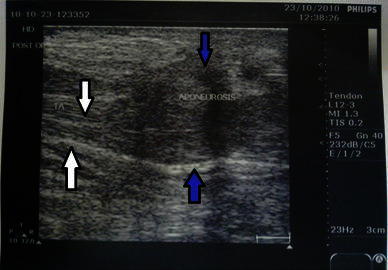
Ultrasound scan after 6 months showing continuation of fibers at the repaired site. The *blue arrows* show the repaired apenurosis, and the *white arrows* show intact tendon
